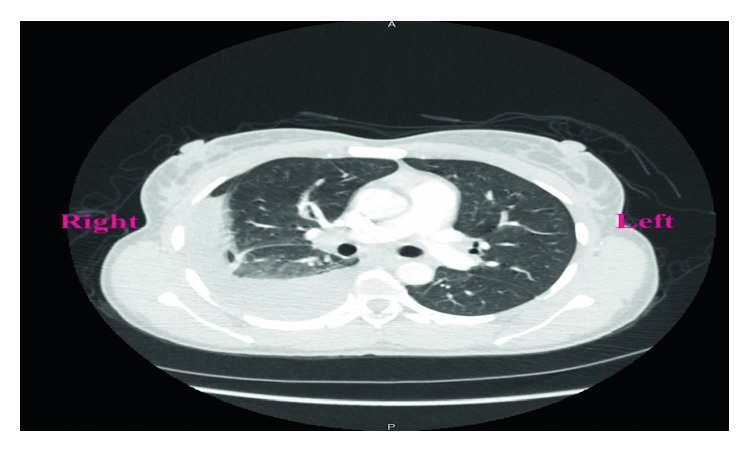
Computed tomography of the chest without contrast. Moderate right pleural effusion with passive atelectasis of the right middle and lower lobes; bilateral hilar lymphadenopathy; perihepatic ascites.
